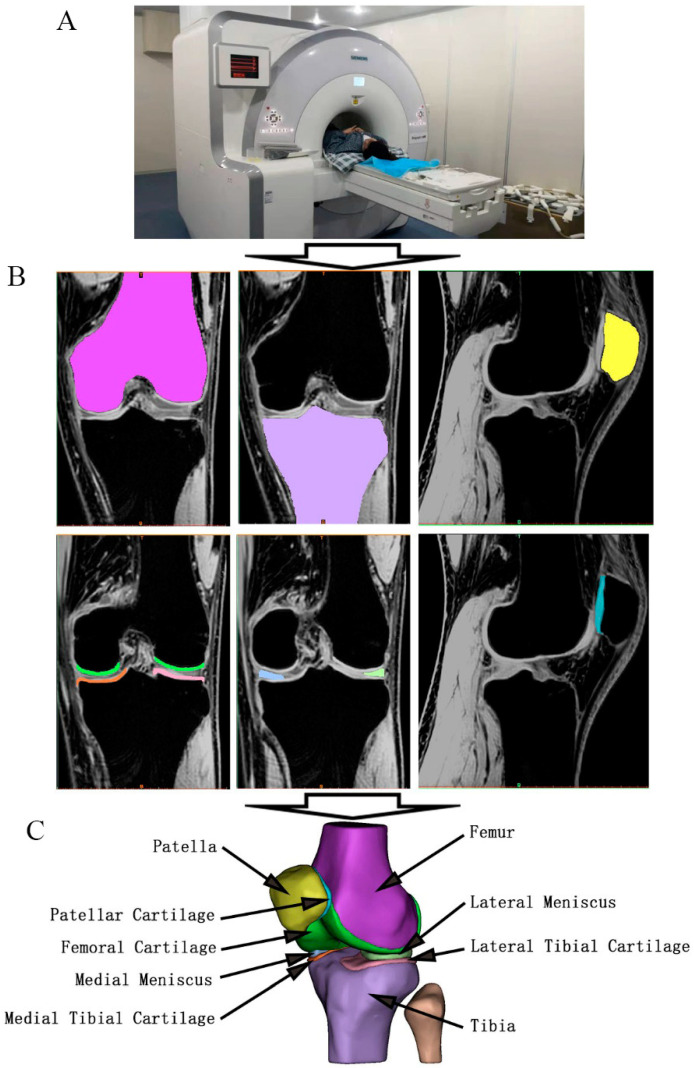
The 3D geometric modeling of bones, cartilages, and meniscus, (A) the MRI scanner, (B) the raw tomographic images, and (C) the 3D geometric models.
